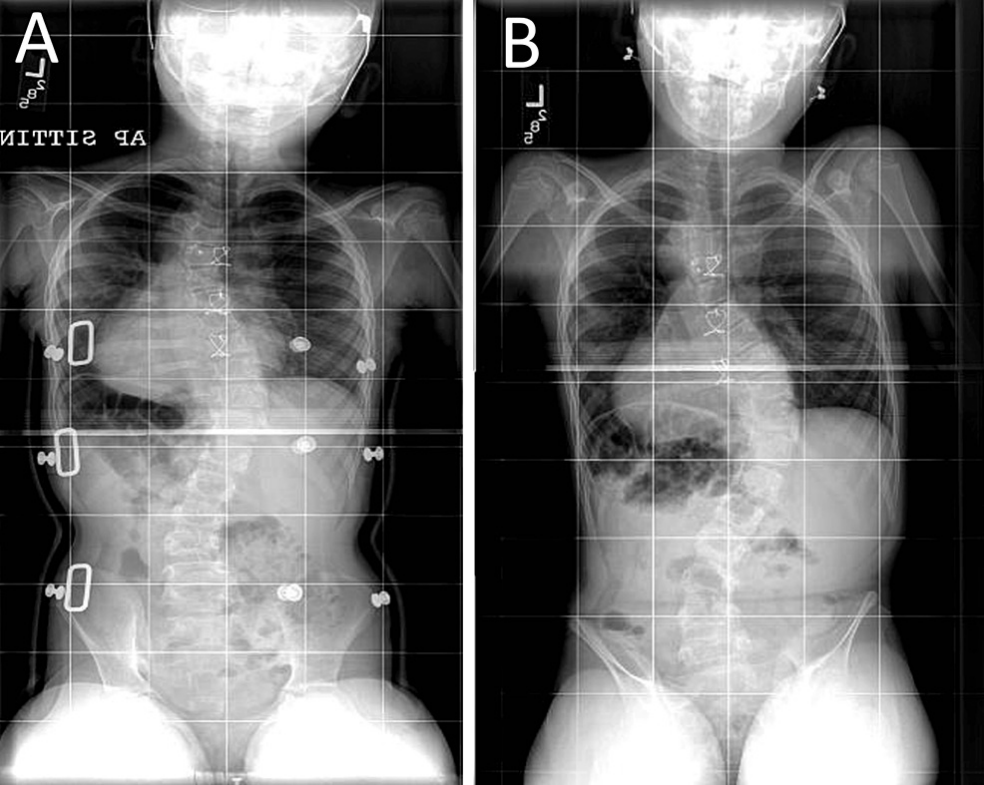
Case three imaging with and without bracing. Imaging with and without bracing. Seated X-rays in A and out B of brace demonstrating progression of the curvature out of brace. Of note, in-brace images were obtained six months before out-of-brace films.
